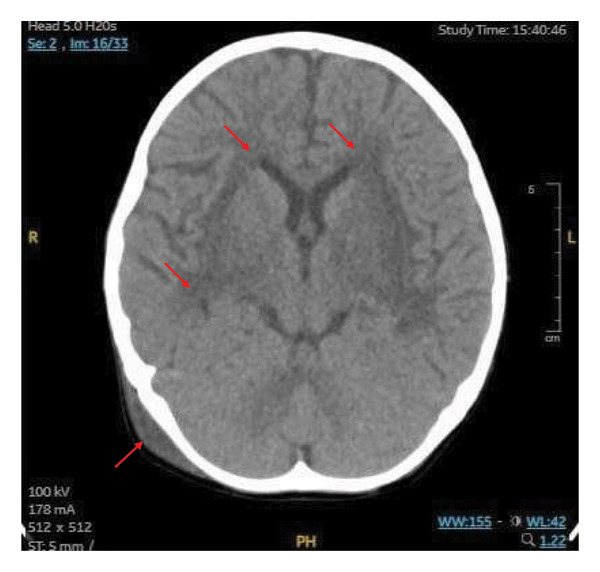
CT scan showing subcortical and periventricular hypodensities in the frontal and parietal lobe white matter involving the lentiform nucleus and internal capsule. There was a small subgaleal swelling over the right parietal bone.
